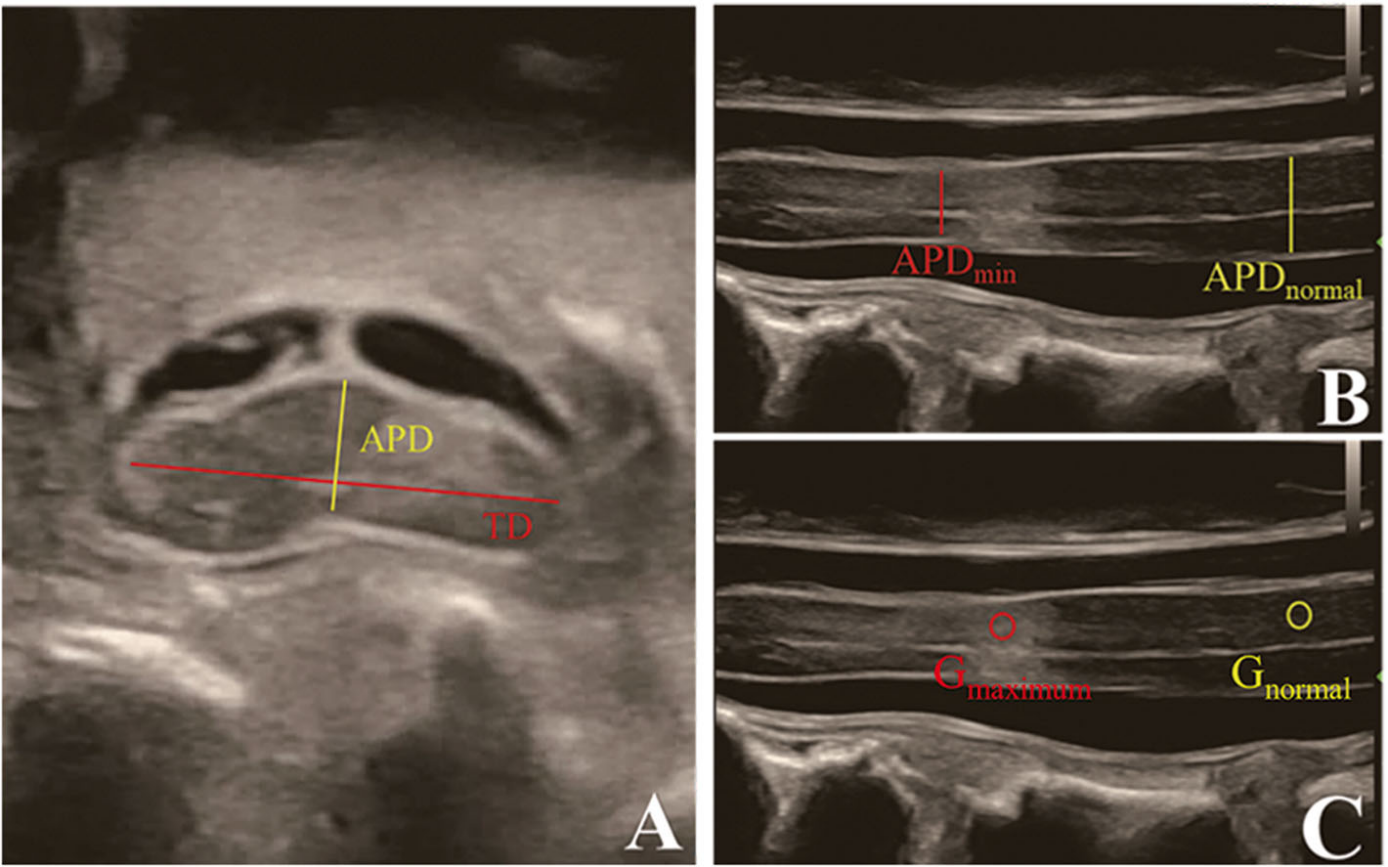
a Traverse images at the maximum level of patient with degenerative cervical myelopathy (DCM), depicting the measurements required to calculate the compression ratio (CR). Measurements for CR of intraoperative ultrasound imaging (IOUSI), including the anteroposterior diameter (APD) and the traverse diameter (TD) of the spinal cord. b) Midsagittal IOUSI of patient with DCM, depicting the measurements required to calculate maximum spinal cord compression (MSCC). Measurements for MSCC of intraoperative ultrasound imaging (IOUSI), including the width of the spinal cord at the narrowest site (APD_min_) and the width of the spinal cord at the normal site (APD_normal_). c Midsagittal IOUSI of patient with DCM, depicting the measurements required to calculate the gray value ratio (R_gray_). A midsagittal intraoperative ultrasound imaging (IOUSI) of a patient, the measurements required to calculate the gray value ratio (R_gray_), including a 0.1 cm^2^ measurement of the gray value at the site of the maximum compression level of the spinal cord (G_maximum_) and 0.1 cm^2^ gray value measurements at the lesion-free site (G_normal_)
